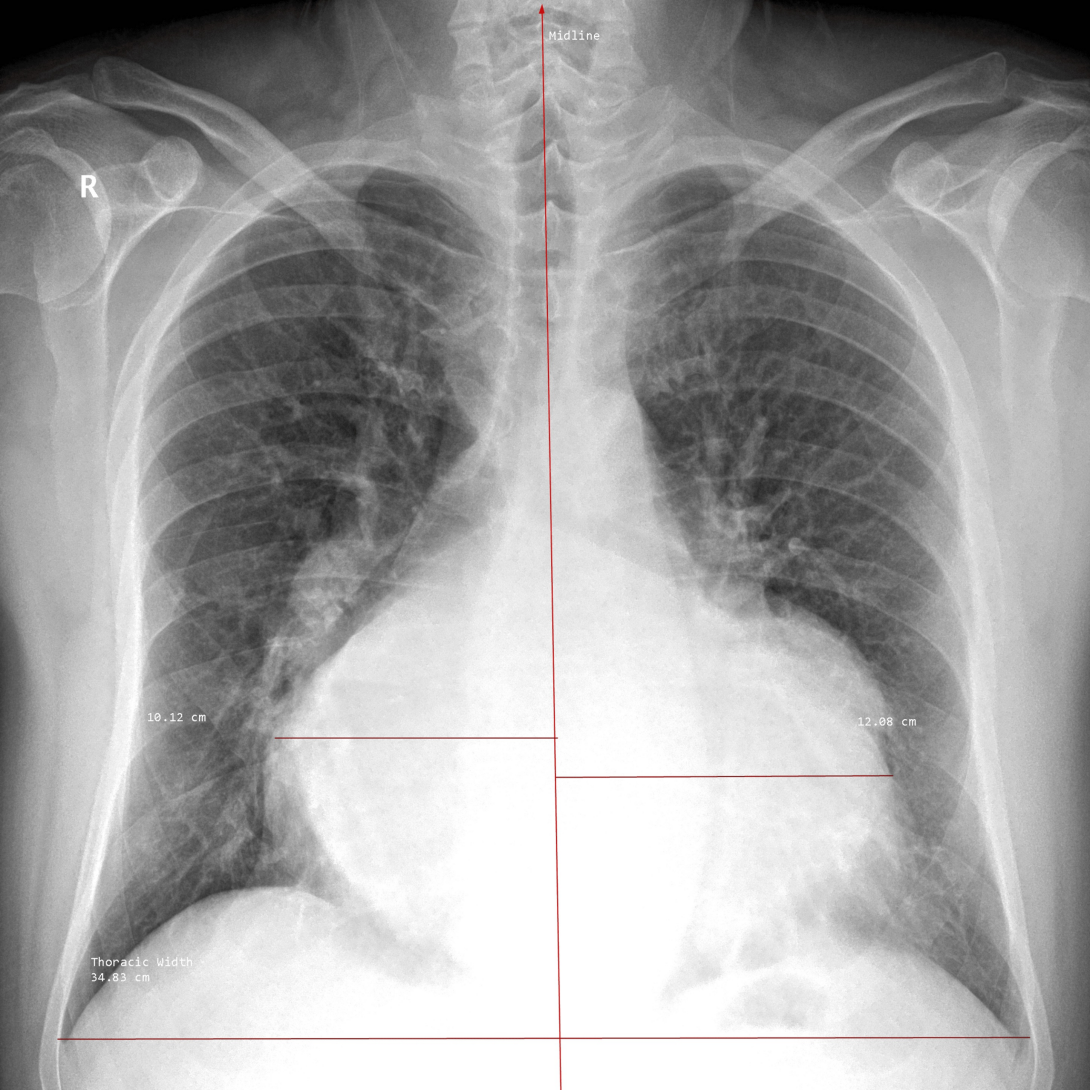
Chest radiograph Posteroanterior chest radiograph demonstrating marked cardiomegaly secondary to massive left atrial enlargement, with splaying of the carina and a cardiothoracic ratio (CTR) of 0.64.
